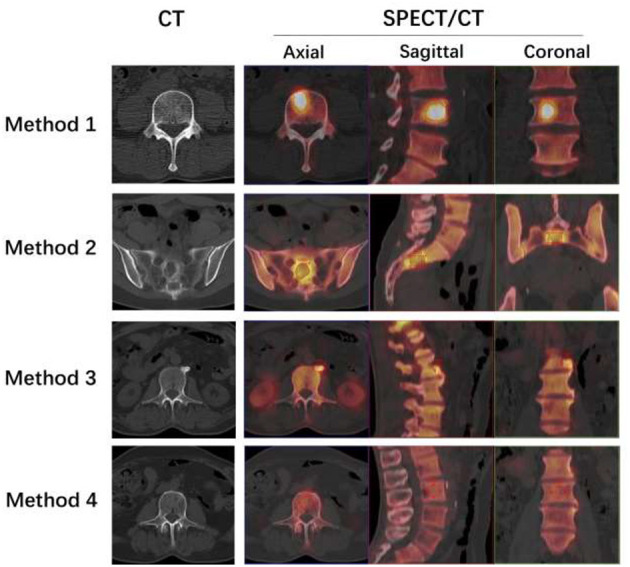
Four methods to delineate different VOIs. Method 1: The L4 bone metastatic lesion was delineated by putting the tumor VOI at 40% of the SUVmax on the SPECT/CT fusion images of axial, sagittal, and coronal sections. The axial section of CT showed no abnormal density changes. Method 2: The Siemens “Multi-frame Polygon” tool was used to manually draw an osteolytic bone metastatic lesion of the sacrum on axial SPECT/CT fusion sections from the top to the bottom of the lesion. Then, the sagittal and coronal images were automatically delineated. The axial section of CT showed osteolytic bone destruction of sacrum. Method 3: On axial, sagittal, and coronal SPECT/CT fusion sections, the hyperosteogeny lesion of L2 was delineated using the “Ellipsoid” tool. The axial section of CT showed osteophytes of L2. Method 4: The visually normal L3 vertebral body was delineated on axial, sagittal, and coronal SPECT/CT fusion sections with volumes not more than 5 cm^3^ using the “Ellipsoid” tool, excluding the bone cortex. The axial section of CT showed normal vertebrae of L3.
